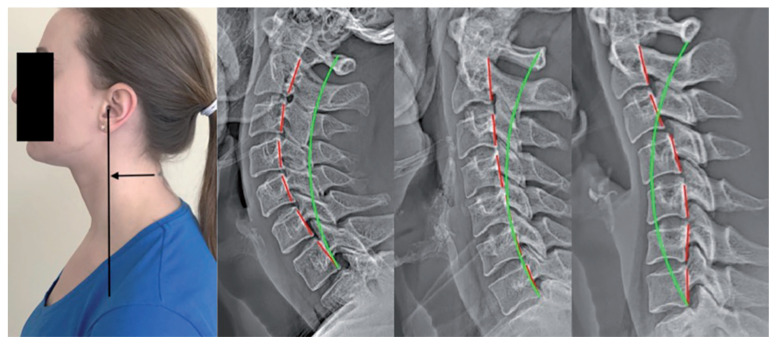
Forward head posture as shown in a posture photograph and three unique lateral cervical radiographs. All three X-ray images have about 25 mm of forward head translation, as measured with the C2–C7 SVA. Left radiograph: hyperlordosis; middle radiograph: hypolordosis; and right radiograph: kyphosis. The green line is a standard alignment; the red line highlights the patient’s alignment along the posterior body margins of cervical vertebra C2 through C7.
